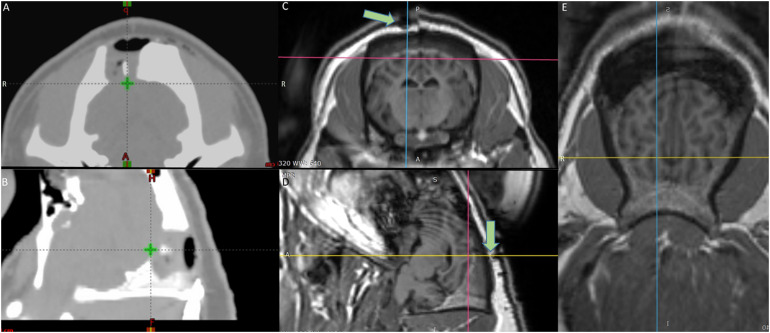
Target definition by the fused MRI/CT (A) axial and (B) sagittal view CT scans of the radiotherapy planning system – The green cross shows the target area on the visual cortex (V1) irradiated with 100 Gy. (C) Axial (D) Sagittal (E) Horizontal view MRI images of the brain – the crosshair shows the target area – Green arrows in (C,D) point at the fiducial marker on top of the irradiated area.
